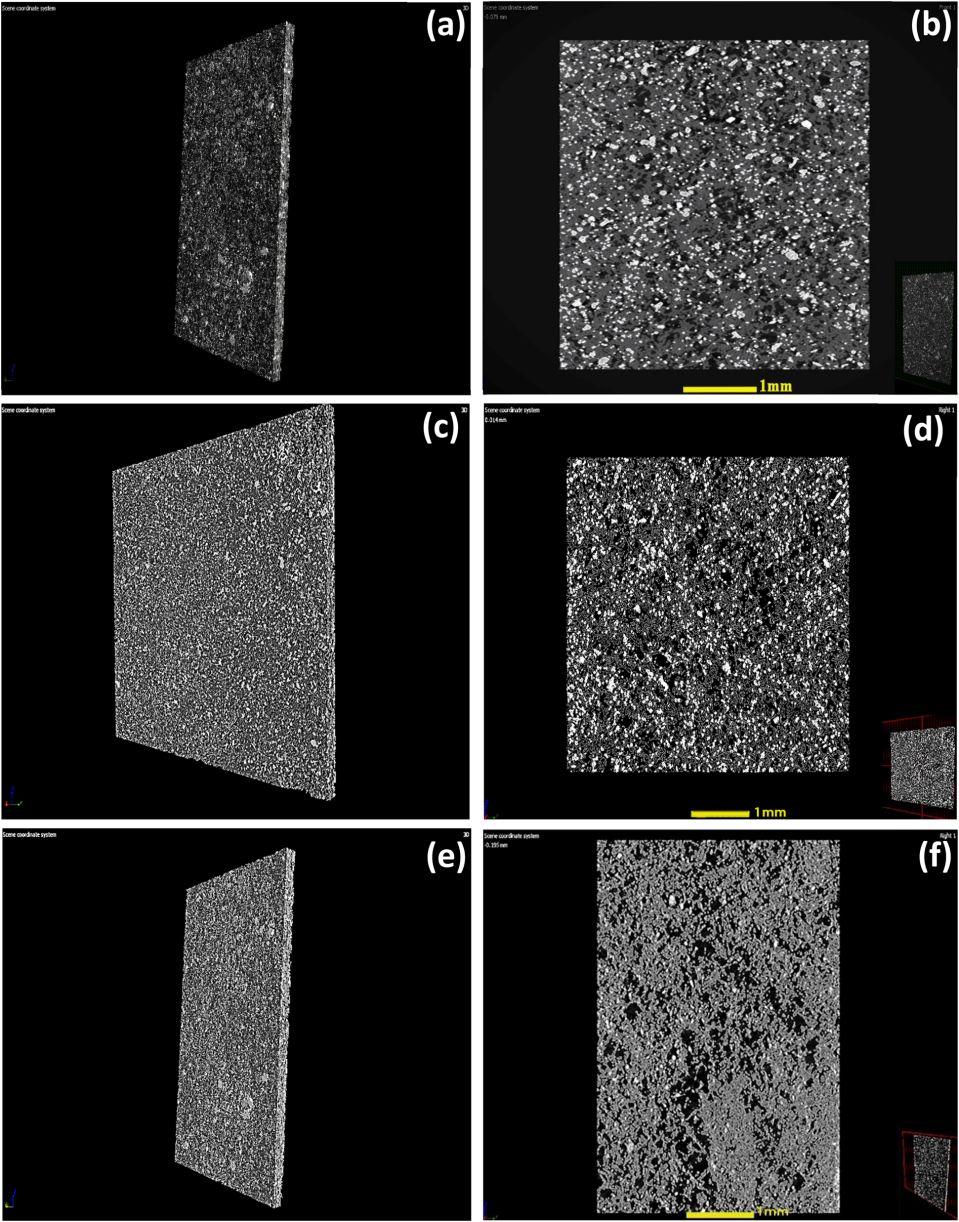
Three-dimensional computed tomography (CT) cross-section analysis of hybrid membranes: WO_3_ NWs–cellulose (a and b), WO_3_ NW–Fe_2_O_3_–cellulose (c and d) and WO_3_ NW–CuO–cellulose (e and f).
